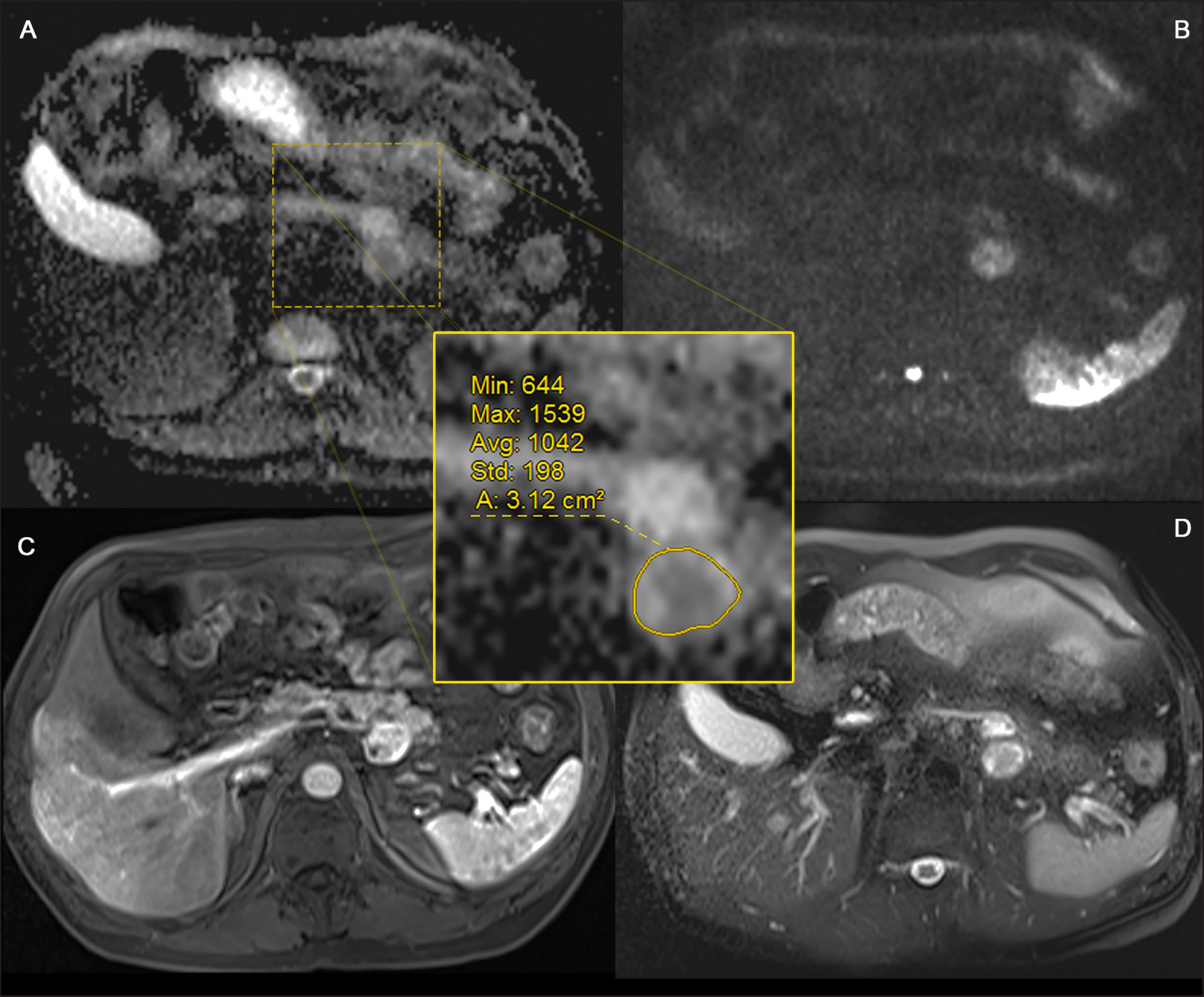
ROI placement (grade 2). Axial ADC map (A), DWI b1000 (B), arterial phase T1- weighted (C) and fat suppressed T2-weighted (D) images of a biopsy proven G2 NET in a 46-year-old male. ROI placement with exclusion of the outermost border of the lesion to avoid artefacts.
